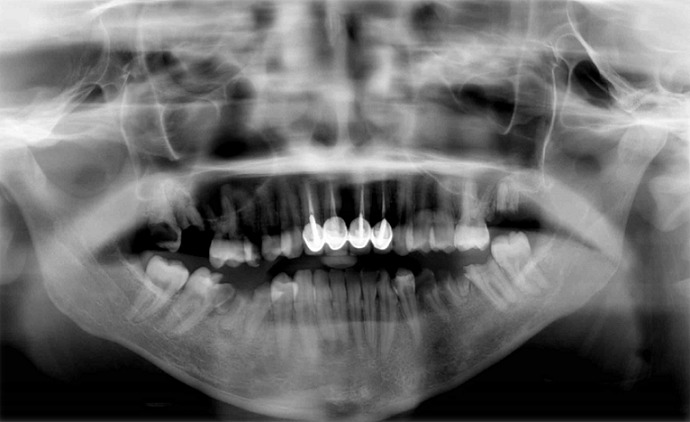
digital panoramic radiograph reveals multiple fixed prosthodontic restorations and multiple carious teeth, multiple remaining roots
